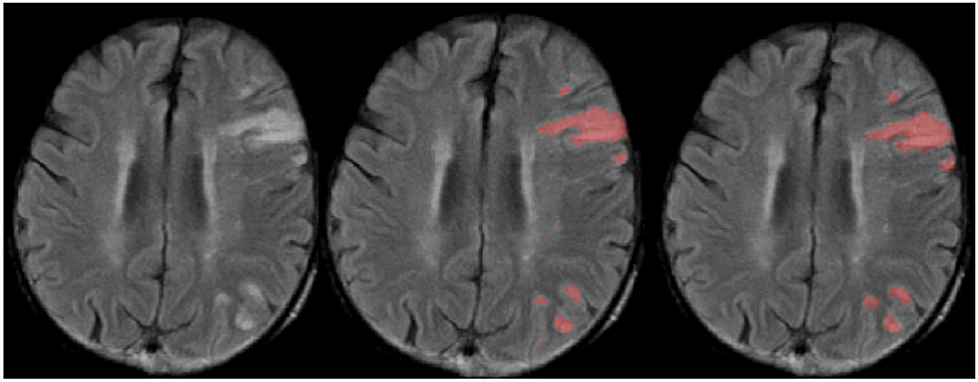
Selected slice from a FLAIR MRI dataset with multiple lesions (Left) and corresponding manual (Center) and automatic lesion segmentation (Right).
